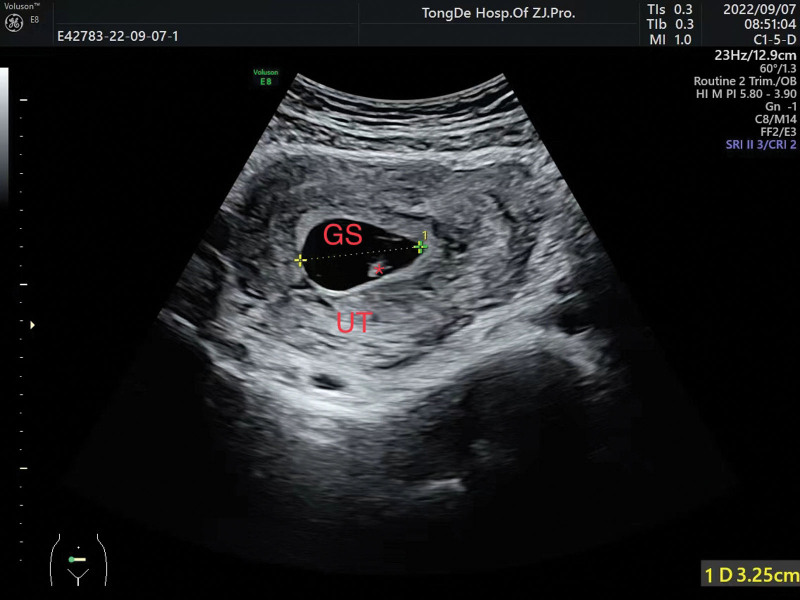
Gestational ultrasound after 8 days of combined treatment (7 weeks, 4 days).
